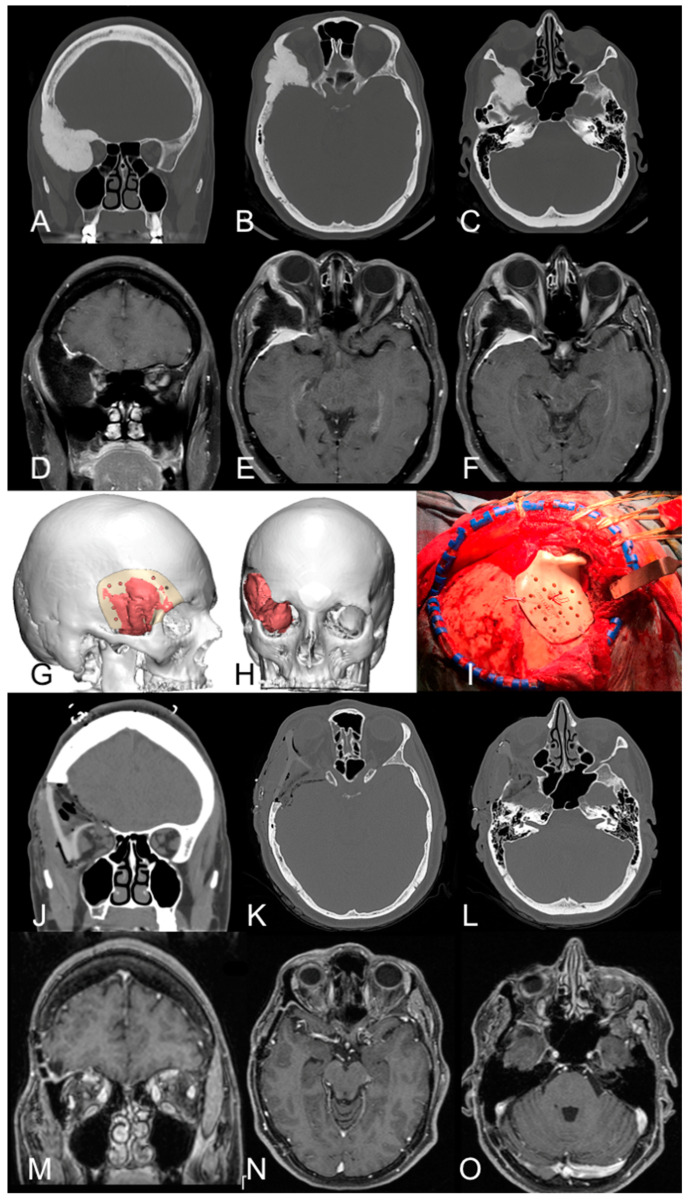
Pre- and postoperative neuroradiology; surgical planning and intraoperative image. (A–F). Coronal (A), and axial (B,C) CT scans and brain MRI T1-weighted sequences with contrast (coronal—(D); axial—(E,F)) show the right spheno-orbital meningioma with the typical features of hyperostosis at the level of the superior and lateral walls of the orbit, and sphenoid wing, with the tumor-induced remodeling of the middle cranial fossa. The lesion caused compression of the orbital cavity with associated exophthalmos. (G,H) Three-dimensional digital reconstruction of the tumor (in red) and the skull prosthesis based on the patient’s anatomy and tumor extension. (I) Intraoperative image of the prosthesis positioned at the end of the tumor removal with the reconstruction of the pterional region and orbital rim. (J–O) Postoperative coronal (J) and axial (K,L) CT scans and brain MRI three years after surgery (coronal—(M); axial—(N,O)) show complete removal of the lesion and optimal positioning of the prosthesis. The resolution of the pre-operative exophthalmos is also shown.
